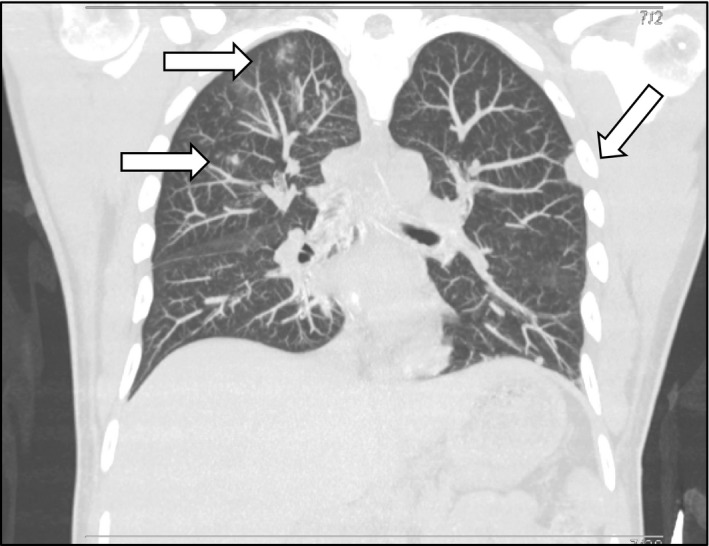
CT Thorax revealing several pulmonary septic emboli (arrows)
